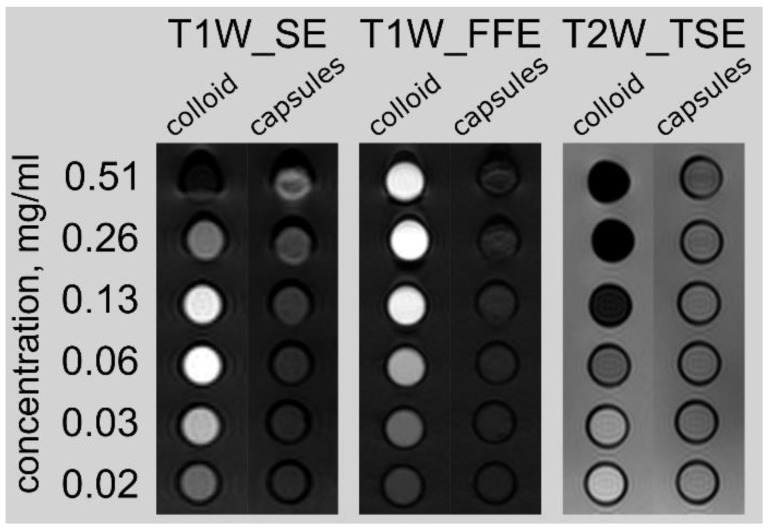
The MRI contrast of the magnetite colloid and magnetic microcapsules at a different concentrations of magnetite in the probe tubes. Different pulse sequences are presented from left to right: T1 weighted “Spin-echo” (T1W_SE), T1 weighted “Fast Field Echo” (T1W_FFE), and T2 weighted “Turbo Spin Echo” (T2W_TSE).
